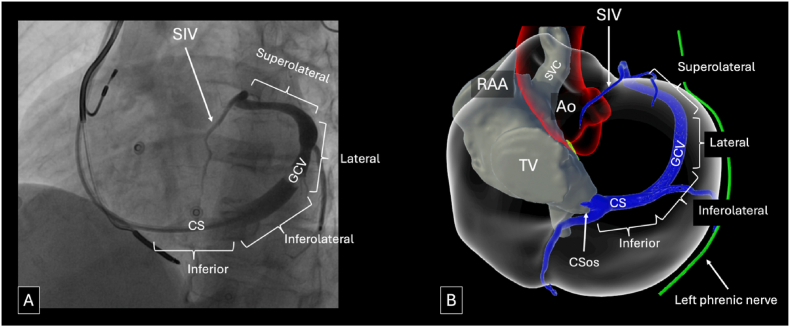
Classification of coronary sinus tributaries in the left anterior oblique (LAO) projection. (A) Balloon occlusion venography demonstrating the coronary sinus (CS), great cardiac vein (GCV), and the superior interventricular vein (SIV, anterior interventricular vein). Tributaries are classified by their position along the atrioventricular groove: superolateral (anterolateral), lateral, inferolateral (posterolateral), and inferior (posterior). The lateral and inferolateral veins represent the primary targets for left ventricular lead placement. (B) Three-dimensional computed tomography reconstruction (inHeart) in corresponding LAO orientation. Coronary veins (blue) and arteries (red) are displayed relative to cardiac chambers. The green line indicates the course of the left phrenic nerve along the lateral pericardium, and its proximity to superolateral and lateral tributaries explains the risk of phrenic nerve stimulation during cardiac resynchronization therapy. Abbreviations: Ao, aorta; CS, coronary sinus; CSos, coronary sinus ostium; RAA, right atrial appendage; SVC, superior vena cava; TV, tricuspid valve. (For interpretation of the references to colour in this figure legend, the reader is referred to the Web version of this article.)
